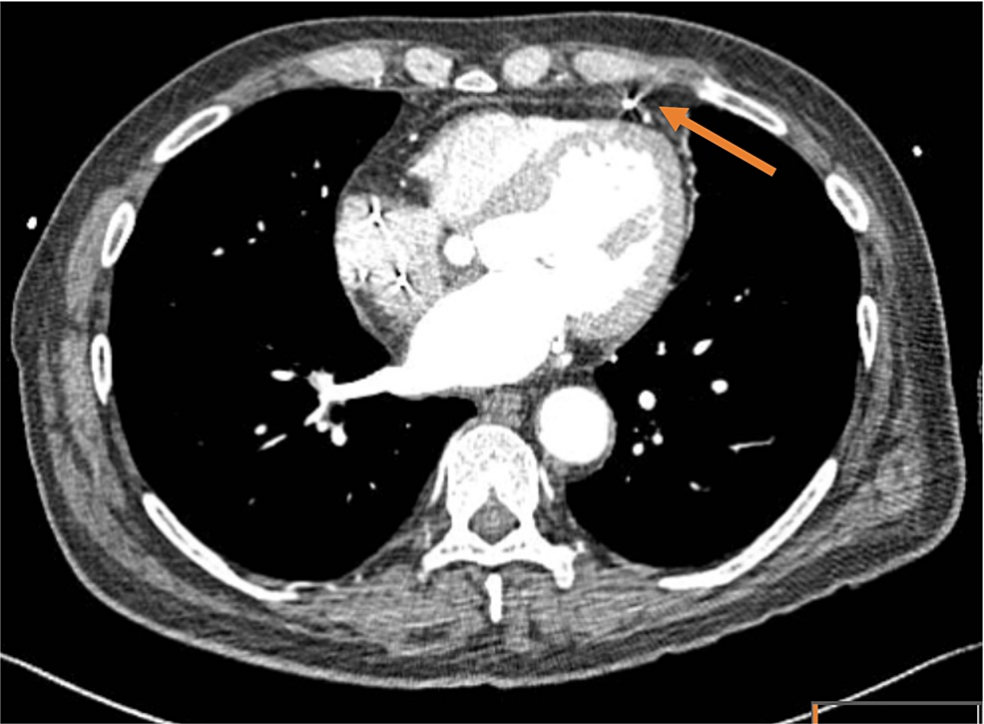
Axial slice of CT chest demonstrating RV lead perforation. RV lead is seen outside of the right ventricle abutting the pericardium. CT: computed tomography; RV: right ventricular.
